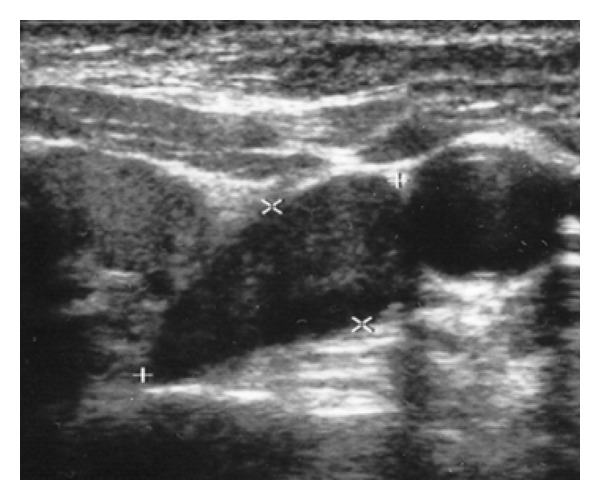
Ultrasound finding of parathyroid carcinoma (transverse)—nonsuspicious “Pad” at the lower pole of the left thyroid lobe.
